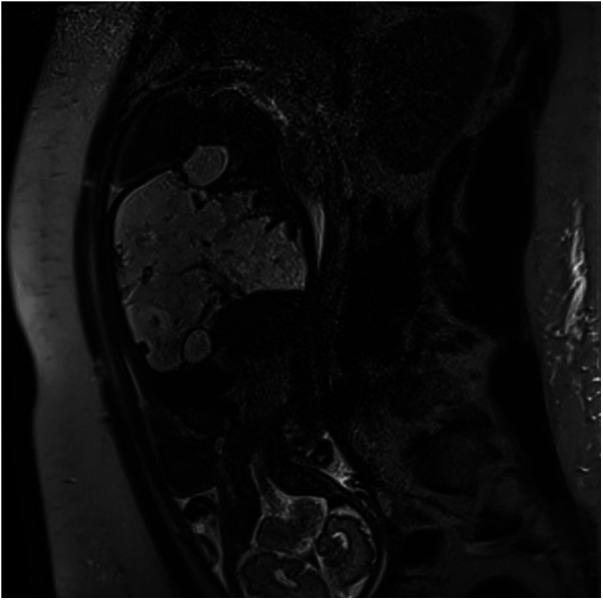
Sagittal MRI scan, T2-weighted sequence, demonstrating the extension of the lesion from the left diaphragmatic dome and the right hepatic margin to the pelvic cavity and transversely across the abdominal cavity (Bambino Gesù Children's Hospital, Rome, Italy).
